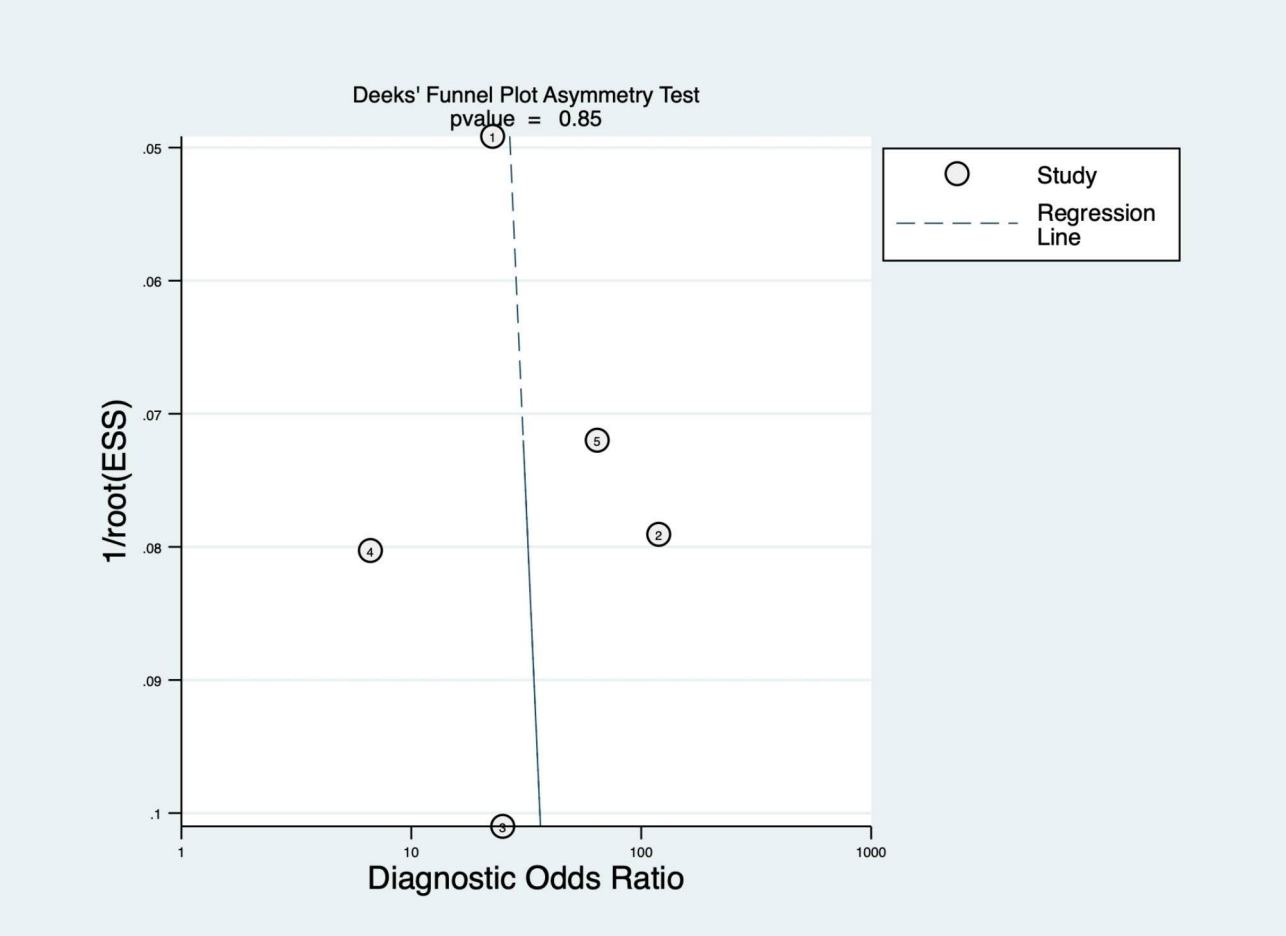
Funnel plot of CT based on artificial intelligence algorithm for predicting lymph node metastasis in breast cancer patients
